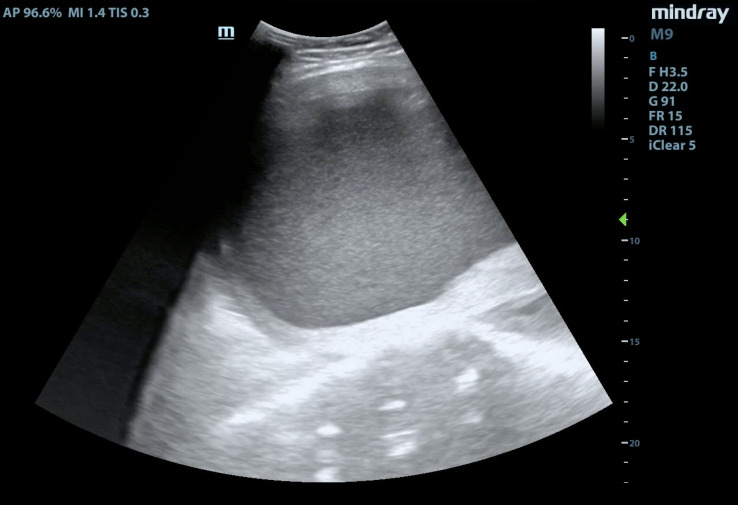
Point of Care Ultrasound Demonstrating an Enlarged Uterus Full of Hypoechoic Fluid in the Longitudinal Plane.
